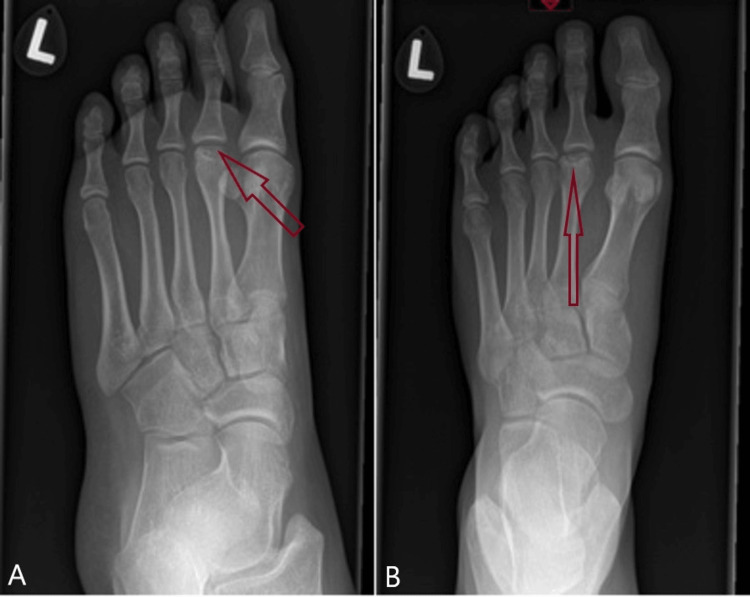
Plain X-rays showing flattening of the head of the second metatarsal are consistent with a diagnosis of Friedberg`s disease A: Oblique view showing flattening of the head of the second metatarsal, consistent with a diagnosis of Friedberg's disease B: Plain AP view showing flattening of the head of the second metatarsal, consistent with a diagnosis of Friedberg's disease
